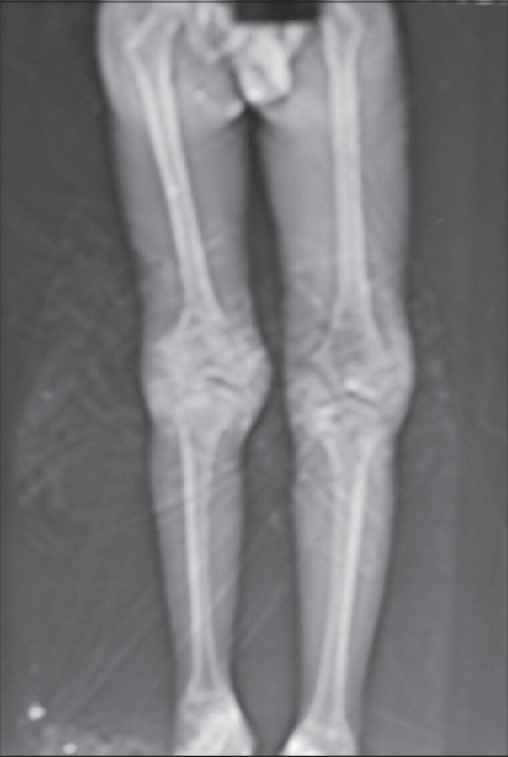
CT scanogram done to determine the limb length and other associated anomalies. There was no limb length discrepancy
